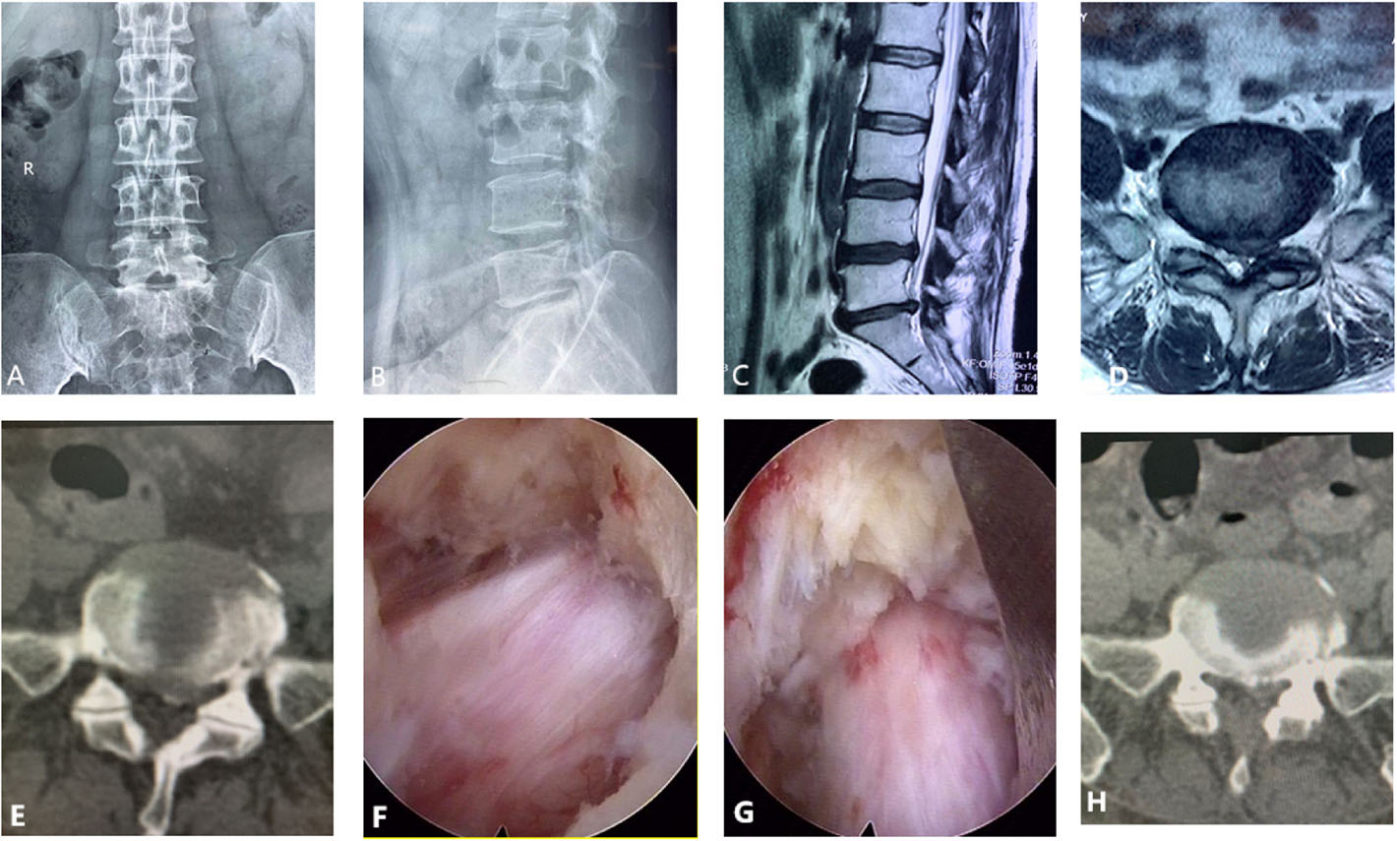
A female patient with lumbar spinal stenosis (LSS) who received PELD. a–e Preoperative X-ray radiography and computed tomography (CT) and magnetic resonance images (MRI) showing severe lateral recess stenosis and hypertrophy of the articular process and lumbar disc herniation with LSS at the left L4-5 level. f, g Intra-operative endoscopic images showing the medial side of the facet and osteophytes have been removed, and the neural tube has been completely decompressed. h Postoperative CT images showing the dorsal partial hypertrophied facet joint and ventral extruded disc had been removed
